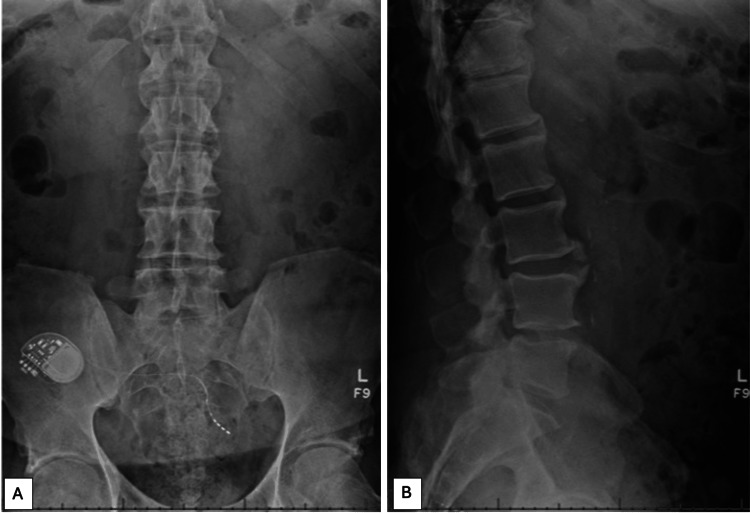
Plain film standing radiographs of the lumbar spine obtained several months after lumbar decompression, as neurogenic claudication symptoms returned. No obvious destructive or expansive osseous lesions were readily identifiable. Figure 5A demonstrates an anterior-to-posterior radiograph of the lumbar spine, while Figure 5B demonstrates a lateral radiograph.
